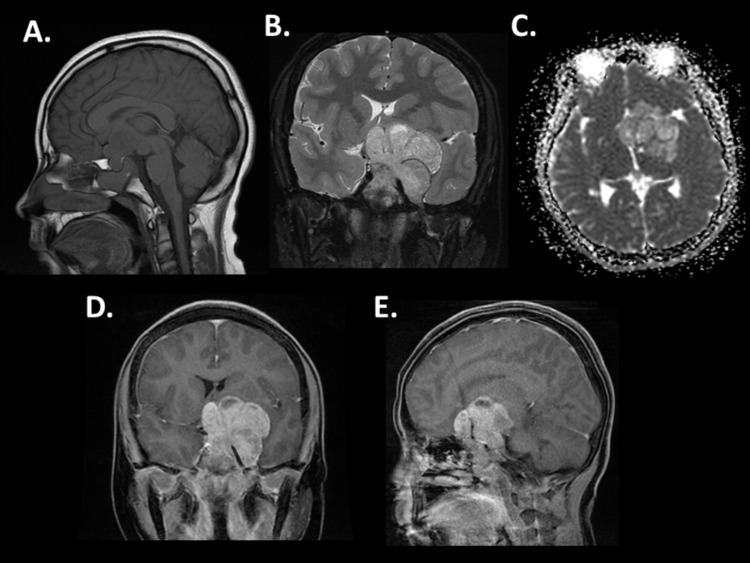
Neuroradiographic features of giant prolactinoma (A) Sagittal non-contrast MRI reveals a large suprasellar mass with obscuration of the optic nerve. T-2 weighted MRI shows extensive lateral invasion (B) and no evidence of reduced diffusivity on apparent diffusion-weighted coefficient sequences (C). Post gadolinium images reveals homogeneous signal with encasement of the cavernous carotid artery (D-E).
